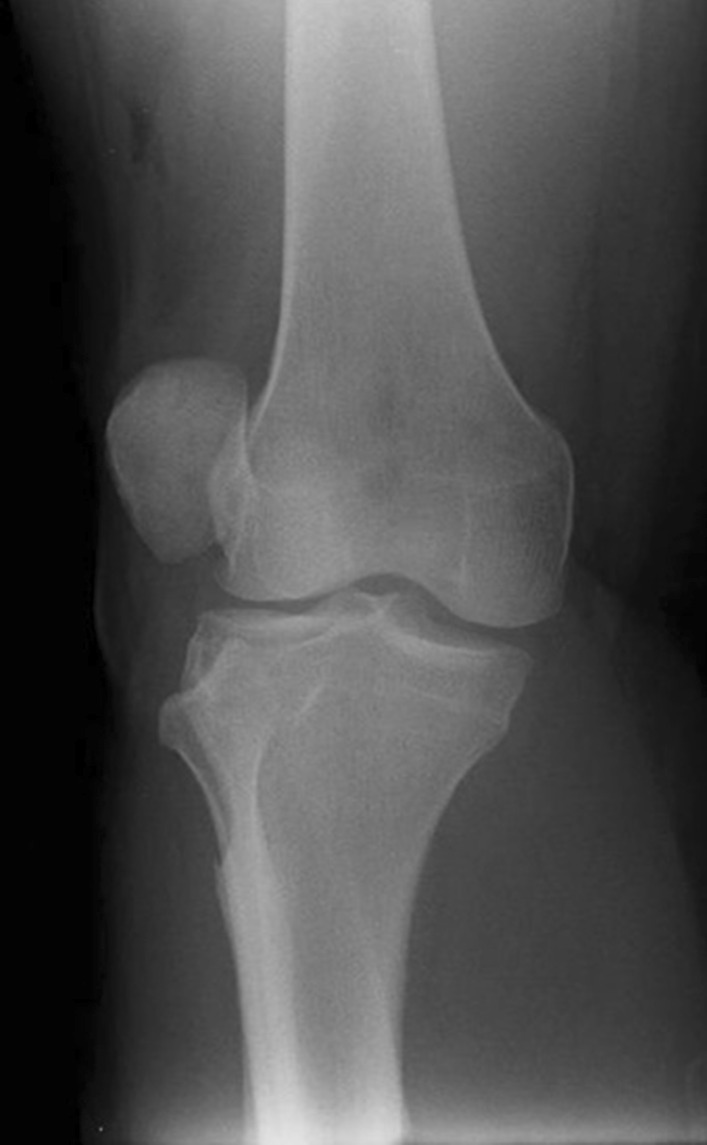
Anteroposterior (AP) view radiograph upon presentation
